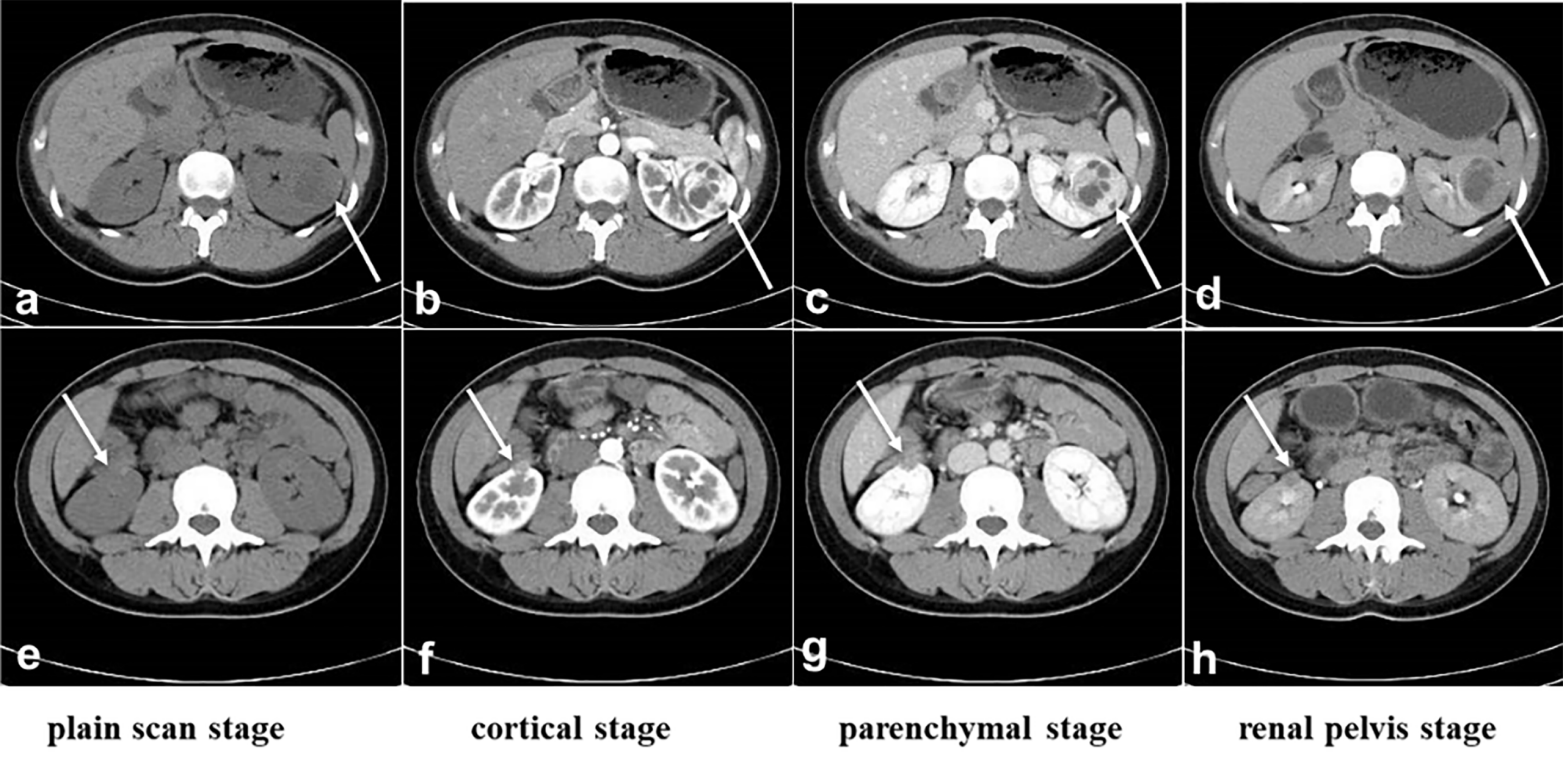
CT before surgery. (A-D): The location and size of the tumor in the left kidney. (E, F): The location and size of the tumor in the right kidney. (A) and (E): Plain scan stage, (B, F): cortical stage, (C, G): parenchymal stage, (D, H): renal pelvis stage. The white arrow indicates the tumors.
